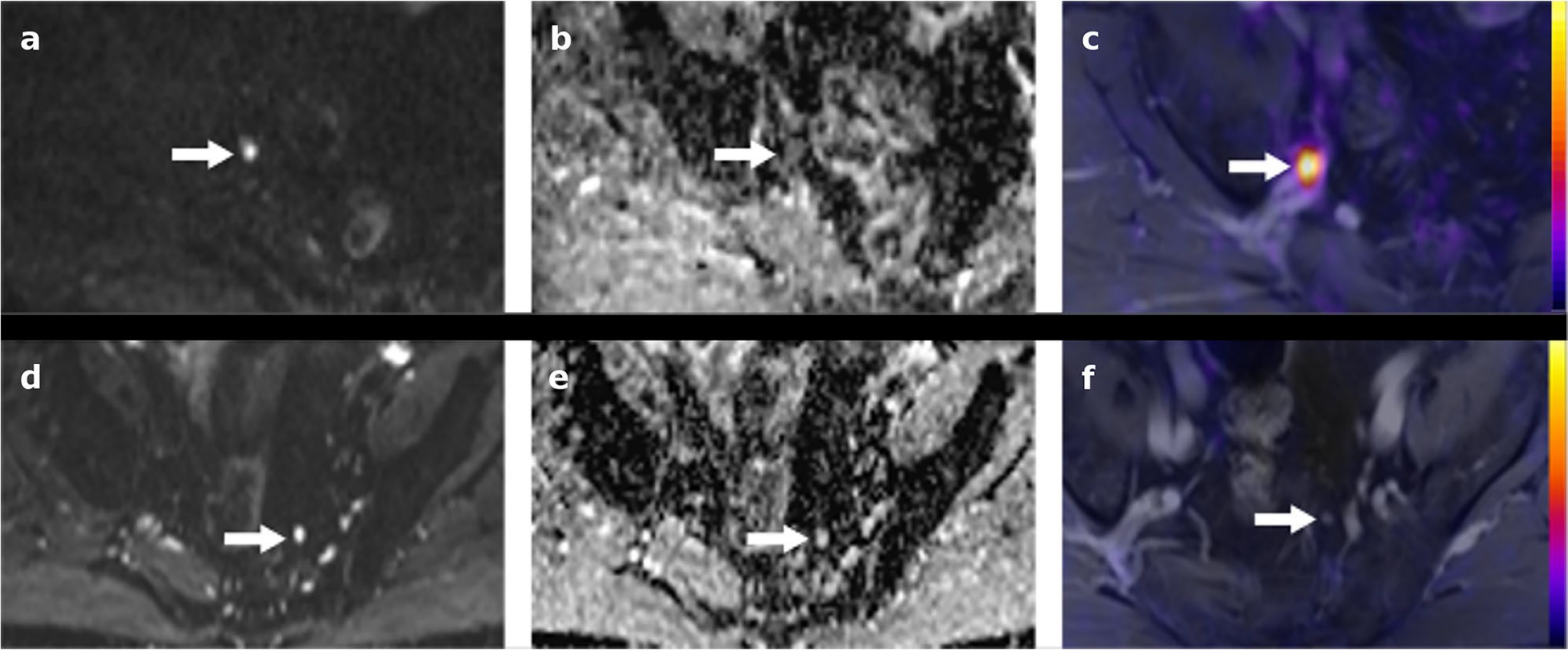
a Focal area of high signal intensity on *b*800 DWI image, corresponding to 6 × 7 mm large lymph node in T1w image (not shown), with low ADC value on the ADC map. b ADC_mean_ 0.81 × 10^−3^ mm^2^/s. c Fusion image of PSMA-PET and gadolinium-enhanced T1 showing strong, focal tracer signal (PSMA+). d In contrast, another focal area of high signal intensity on *b*50 DWI images, e corresponding to a 5 × 6 mm large lymph node, with comparably high ADC value on the ADC map, ADC_mean_ 1.26 × 10^−3^ mm^2^/s). f Fusion image of PSMA-PET and gadolinium-enhanced T1 image showing no apparent tracer signal (PSMA−)
